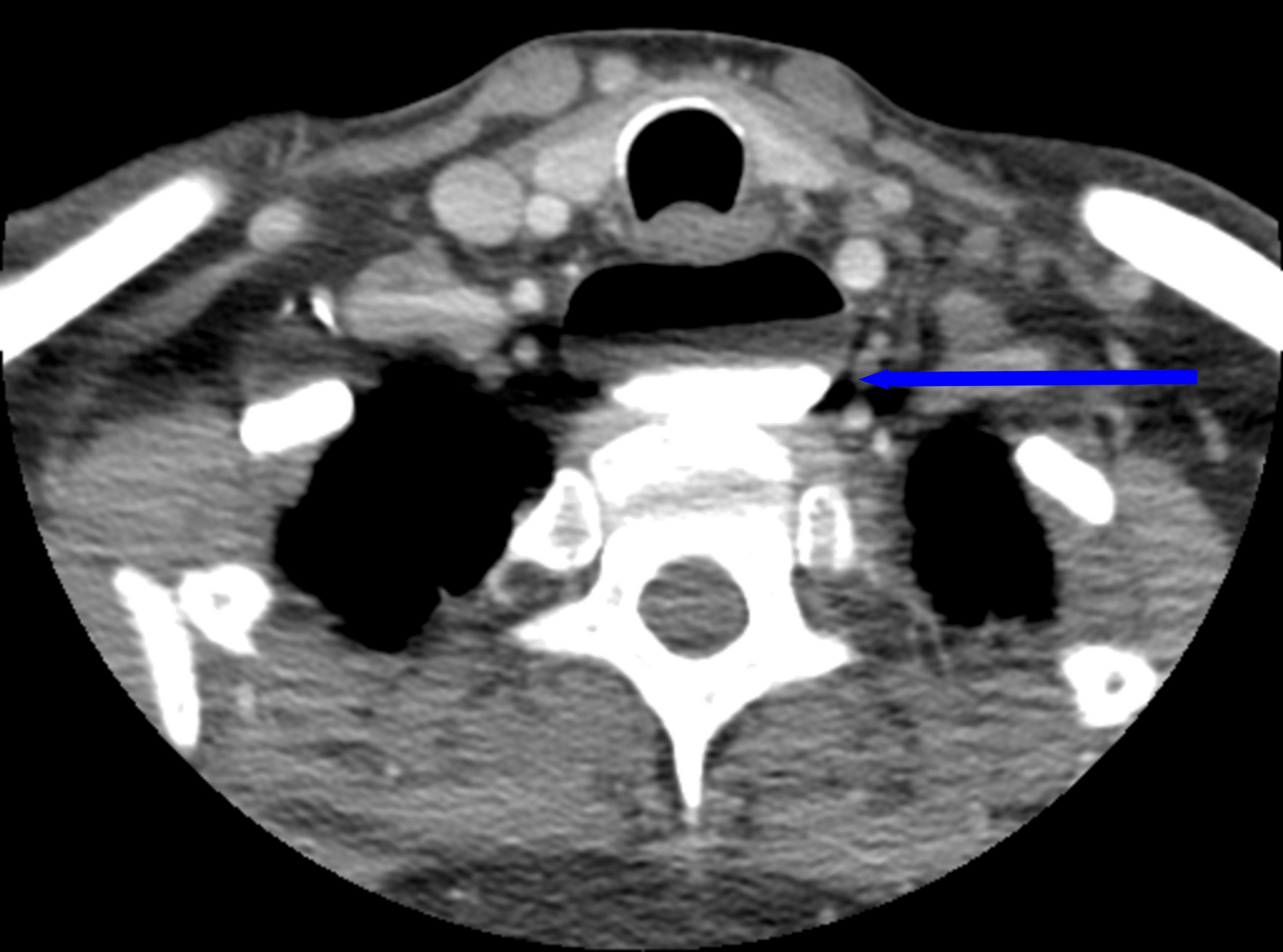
CT soft tissue neck showing a large Zenker’s diverticulum with compression of the proximal esophagus.
